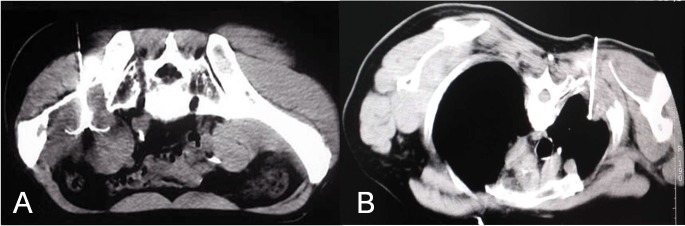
Computed tomography images showing the percutaneous placement of the radiofrequency ablation electrode A. Image of a 57-year-old man with metastatic hepatocellular carcinoma involving the right ilium. B. Image of a 67-year-old man with metastatic non-small-cell lung carcinoma involving the rib.
